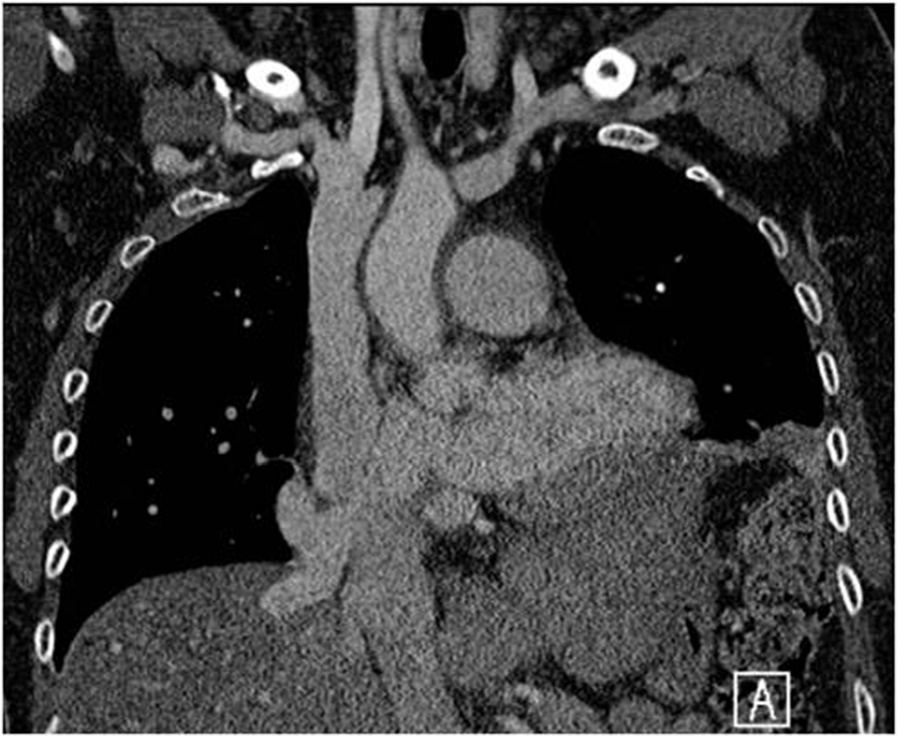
Anomalous drainage of the right hepatic vein. Coronal contrast-enhanced computed tomography scan shows the anomalous drainage of the right hepatic vein within the same orifice as the scimitar vein, redirecting the blood pool of the right hepatic vein into the left atrium. Note the normal drainage of the middle hepatic vein into the inferior vena cava.
